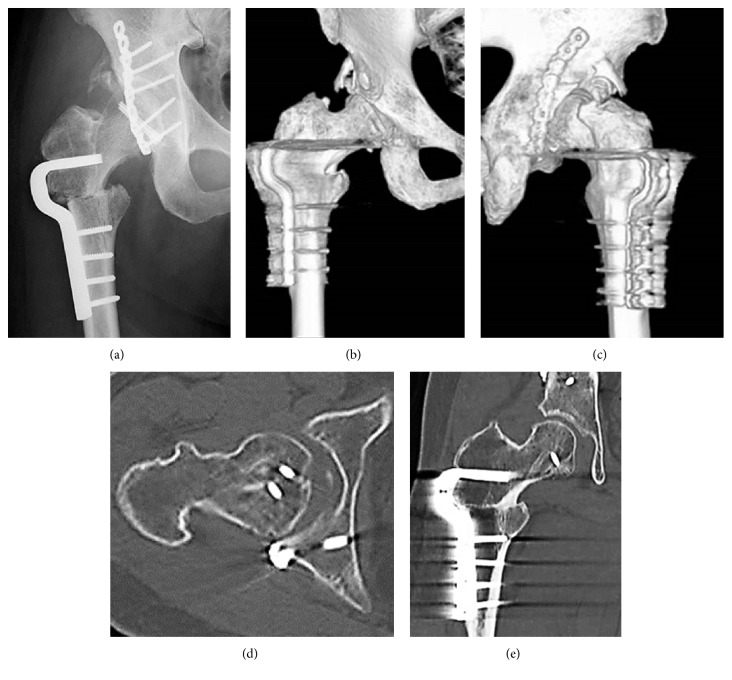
Case 1. (a) Anteroposterior X-ray image of the right hip after a Pauwel intertrochanteric varus osteotomy. (b) Anteroposterior and (c) posteroanterior three-dimensional computed tomography showing derotation of 35° and varus of 30°. (d) Axial image and (e) coronal computed tomography showing congruity was improved and internal fixation was stable.
